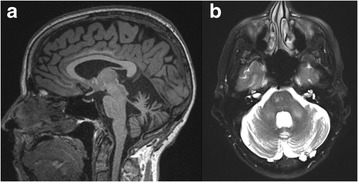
a Sagittal T1 MRI demonstrates severe atrophy of the cerebellar vermis and hemispheres. b Axial T2 MRI demonstrates patchy hyperintensities involving the pons and middle cerebellar peduncles and ex vacuo dilatation of the 4th ventricle
